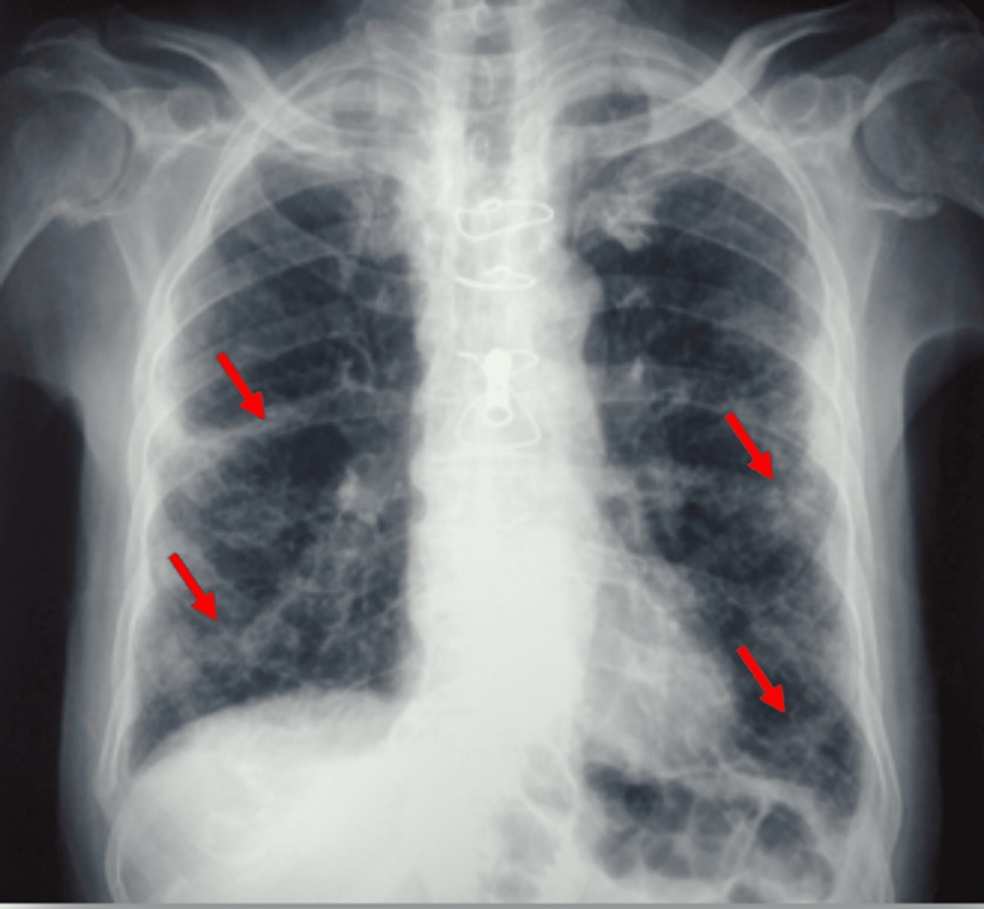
Thorax radiography: interstitial lung disease Presence of reticulo-micronodular interstitial infiltrates (red arrows)
